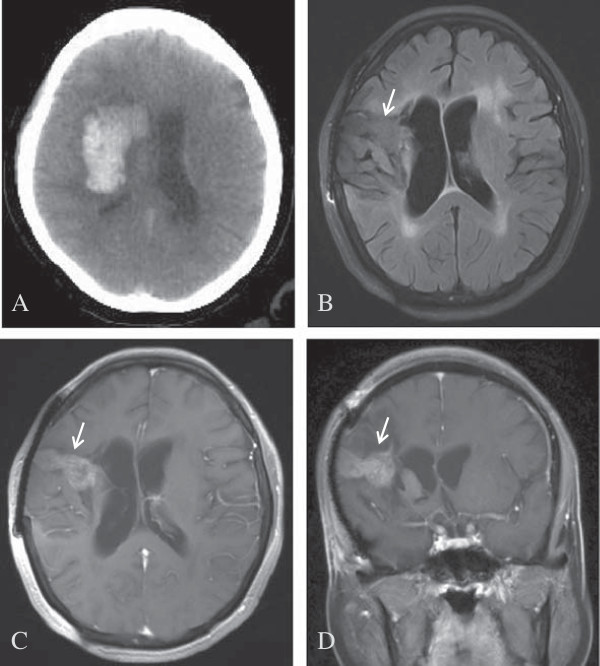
Images taken before and after surgery. (A) Computed tomography (CT) scan showing a region with high-intensity hemorrhage in the right basal ganglia. (B) Magnetic resonance imaging (MRI) results showing irregularly shaped lesions in the right frontal temporal lobe and basal ganglia, with mixed signal and dark fluid with low or higher signal. Lesion size was 1.7 cm × 3.7 cm. (C-D) Lesion observed after strengthening the signal (arrow).
